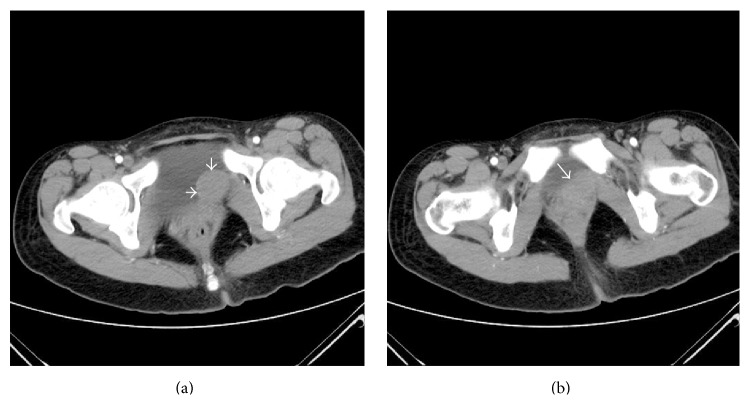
Contrast-enhanced computed tomography (CT). (a) shows a diameter of about 3 cm soft, mild enhancement mass at the left posterior wall of the bladder, protruding into the bladder locally. (b) shows the mass that was adjacent to bladder neck and vagina.
